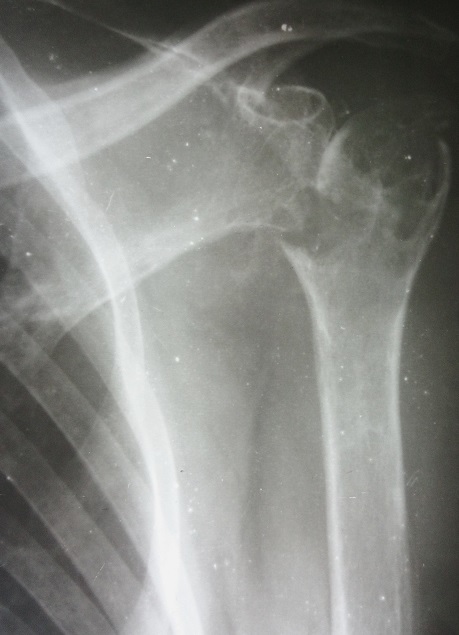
Radiographie de l’épaule de face, image séquellaire d'ostéonécrose septique de la tête humérale
